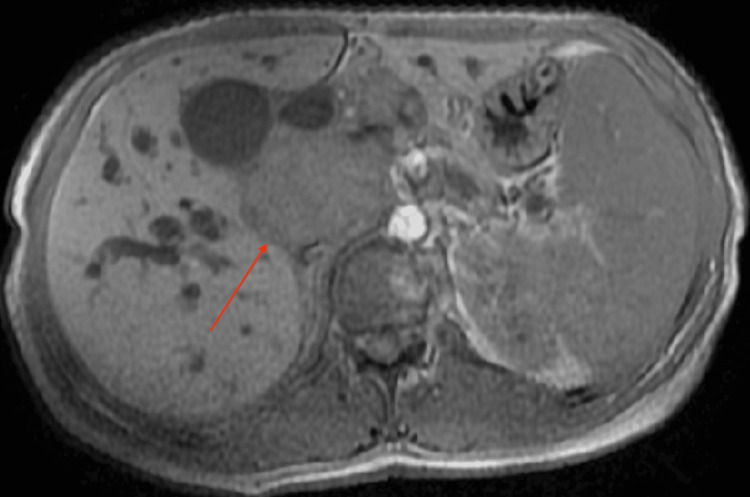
T1-weighted MRCP image showing a periportal lymph nodal mass Axial T1-weighted MR image demonstrates a hypointense conglomerate lymph nodal mass in the periportal and peripancreatic region causing extrinsic compression of the common bile duct.
